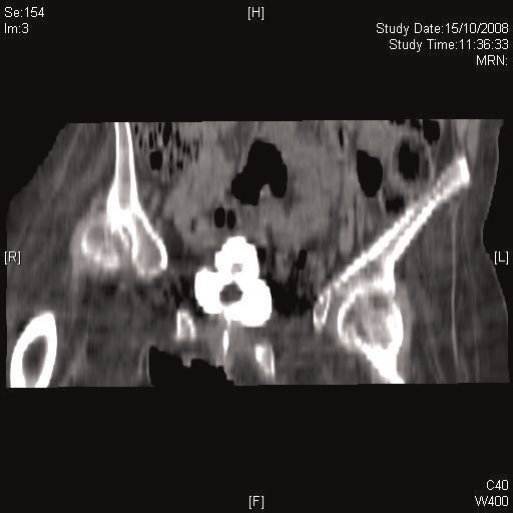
Coronal reconstruction of CT cystography, performed on 15 October 2008, shows hourglass bladder with smaller superior compartment and larger inferior compartment.
